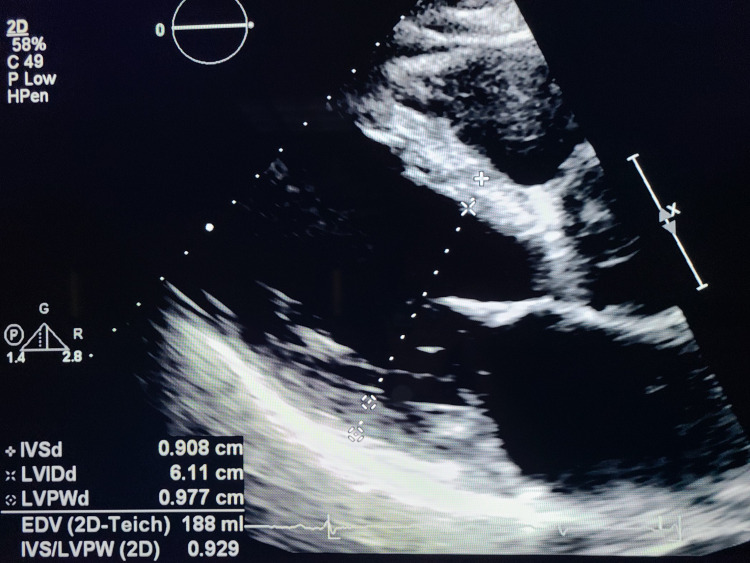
Echocardiography demonstrating aortic regurgitation
